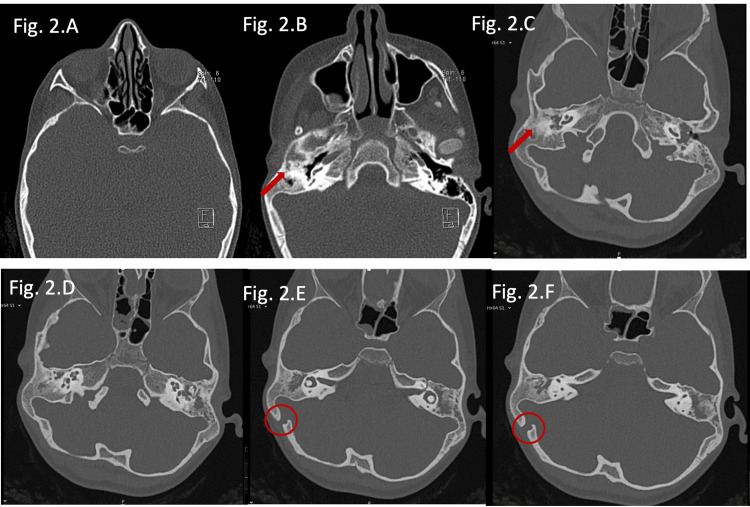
(A-F) Temporal bone CT scan (axial section) of Patient 1. An external auditory canal atresia (red arrow) and microtia can be seen, as well as a middle ear malformation. A very thin skull bone (2A) and a large right emissary vein posteriorly (red circle) are also evident.
